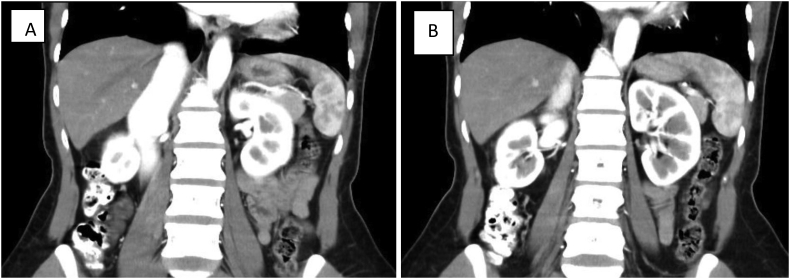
A and B Coronal reconstructions of CT angiography demonesrating flow through the renocaval fistula with contrast seen in the dilated inferior vena cava.
